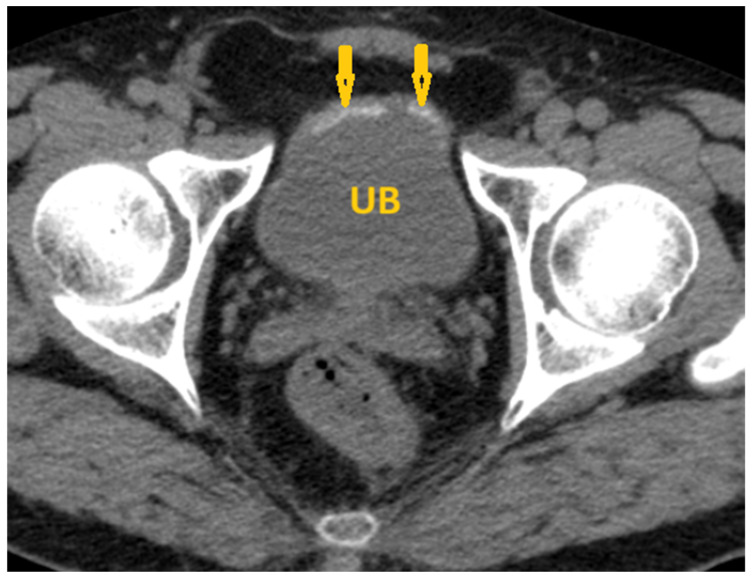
Selected non-contrast computed tomography axial image of a 46-year-old male patient shows mildly thickened urinary bladder wall with linear calcification (arrows) at the anterior aspect, reflecting *Schistosoma* cystitis. UB: Urinary bladder.
